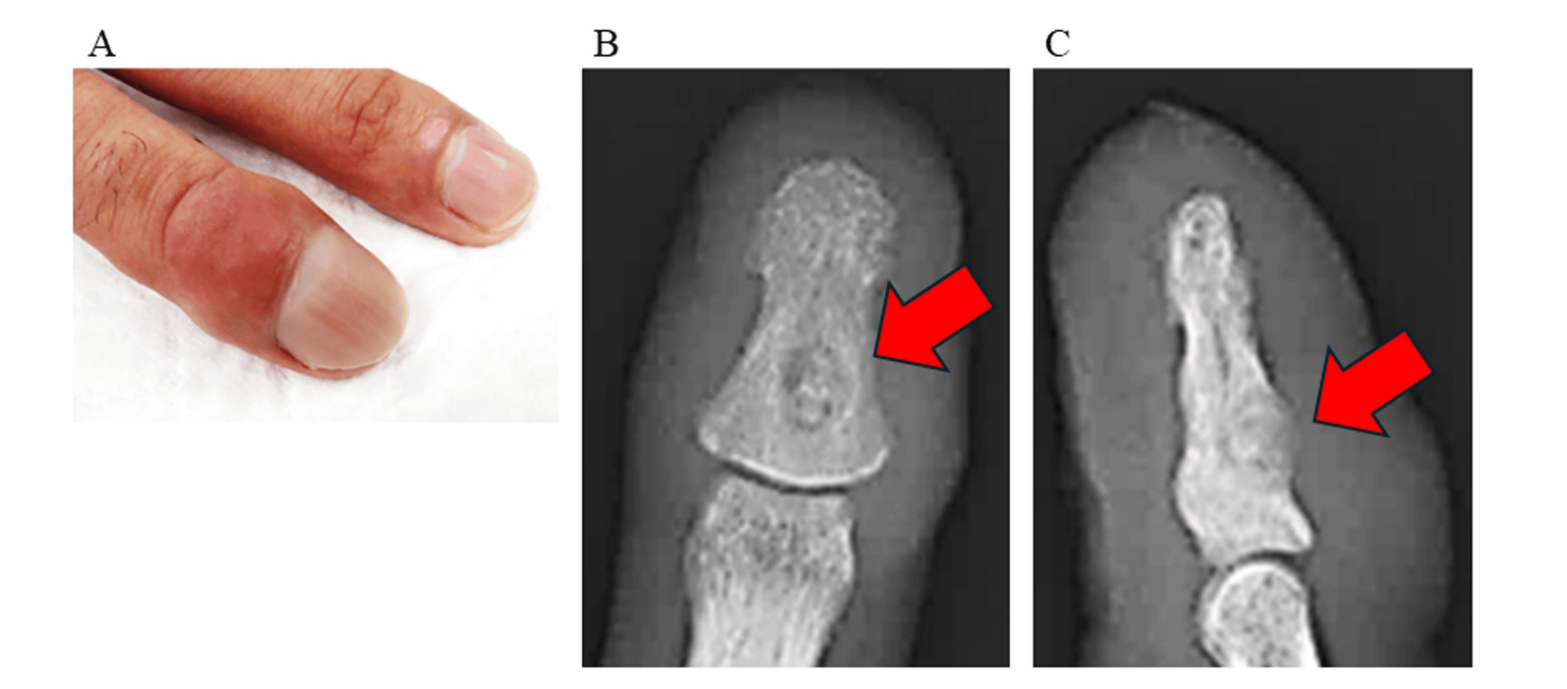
Gross appearance and preoperative radiographs A: Gross appearance of the distal right ring finger showing club-like thickening; B: Preoperative radiograph of anteroposterior view revealing a circular radiolucent lesion with internal calcification in the distal phalanx of the right ring finger (red arrow); C: Preoperative radiograph of lateral view demonstrating the same lesion on the dorsal aspect of the distal phalanx (red arrow)
